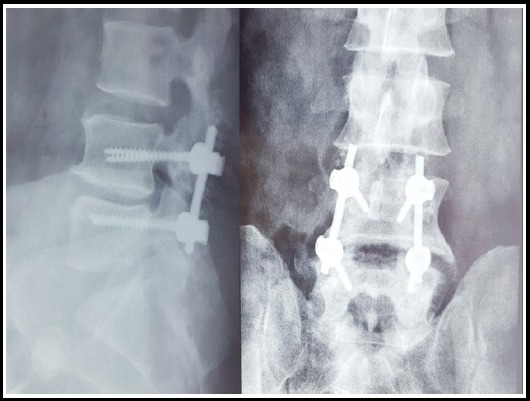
Plain X-ray thoracolumbar spine showing post-operative images of a L4-5 pedical screw fixation for dynamic listhesis.
